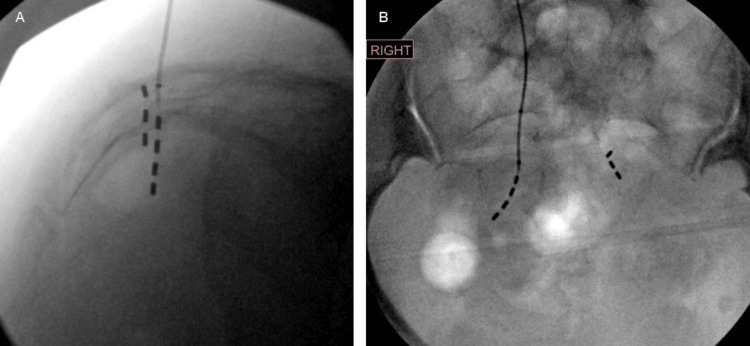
Final pelvic radiographs after lead removal and replacement as well as retained distal lead segment A) Lateral view demonstrates the retained distal lead segment within the sacrum. B) AP view of the new lead on the contralateral side
